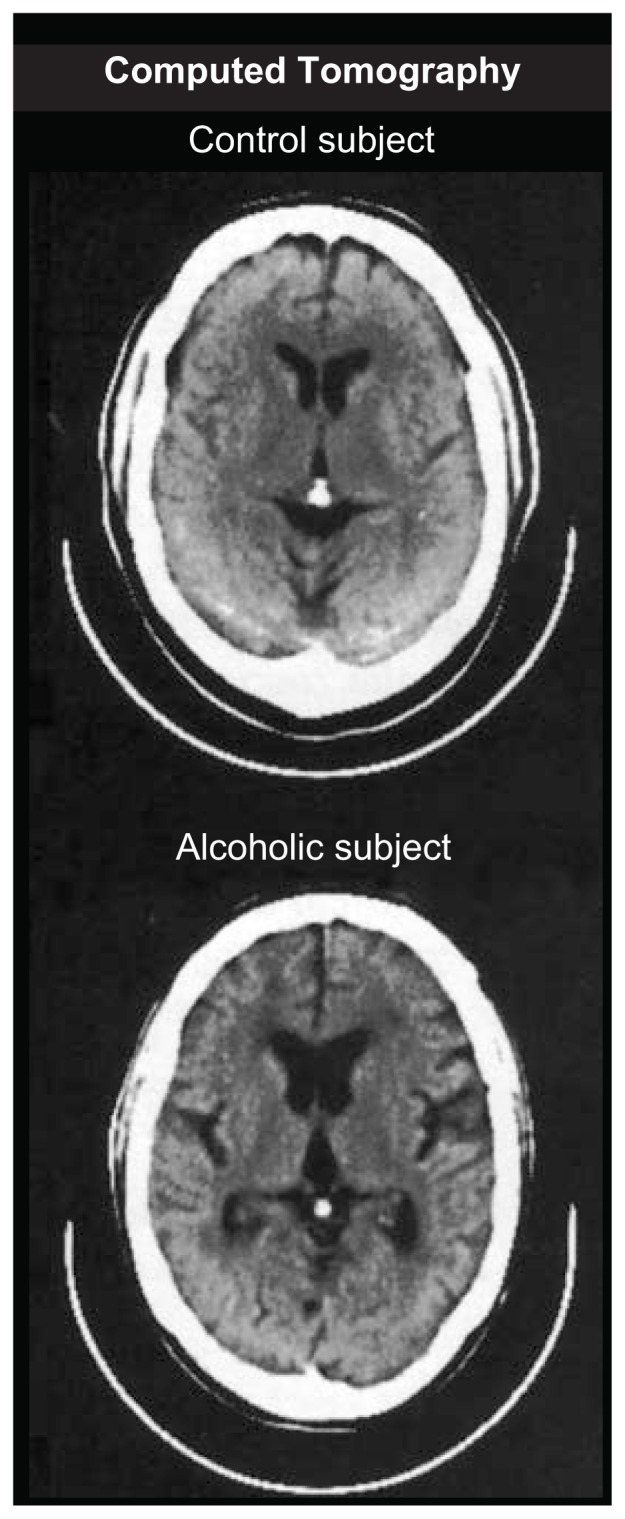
These computed tomography images show loss of brain volume in a 60-year-old alcoholic man compared with a 61-year-old nonalcoholic man. These images depict slices of brain tissue 10 mm thick. Image courtesy of Margaret Rosenbloom.
